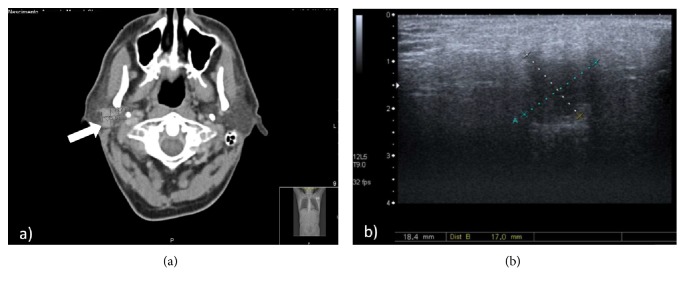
Cervico-thoraco-abdominal CT scan exposed a 16 × 1 × 17 mm nodular image on the right parotid gland (a); Parotid ultrasound documented a non specific 18 mm hypoechogenic nodule in the right parotid gland, with no signs of vascular invasion and no cervical adenomegalies (b).
